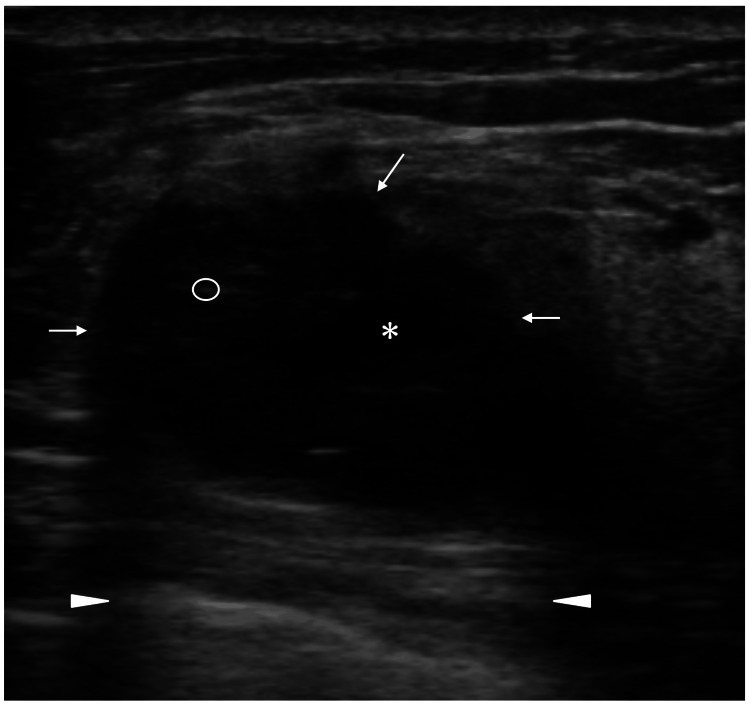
Ultrasound findings Ultrasound showed an oval mass with distinct mass borders (arrows), a focal presumed liquid part (asterisk), faint punctate internal high echoes (circle), enhanced posterior echoes (arrowheads), and very low internal echoes.
